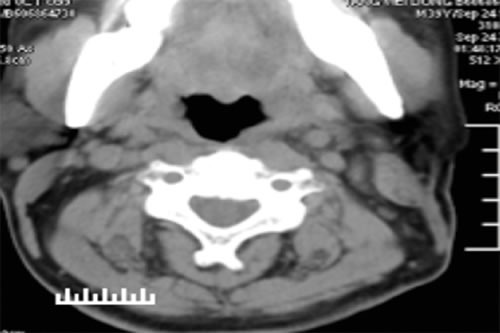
CT scan of the neck 6 months after surgery Note the absence of the left cervical mass and abnormal lymph nodes seen prior to treatment.
